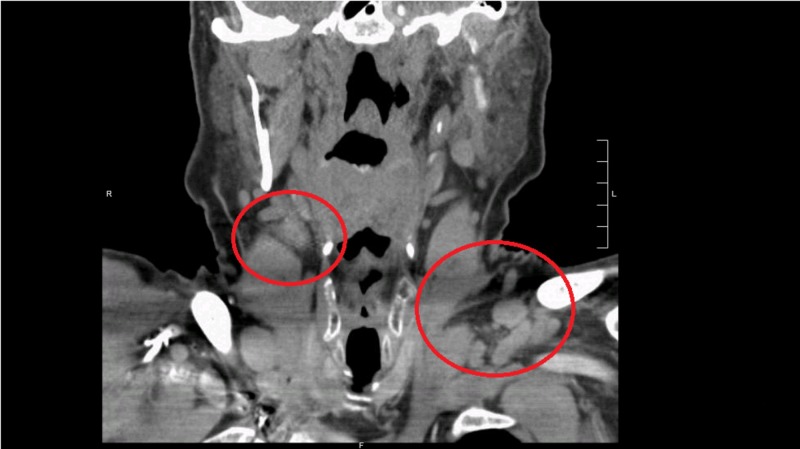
CT scan neck and soft tissue. Diffuse cervical lymphadenopathy along with enlarged clavicular lymph nodes (red circles).
